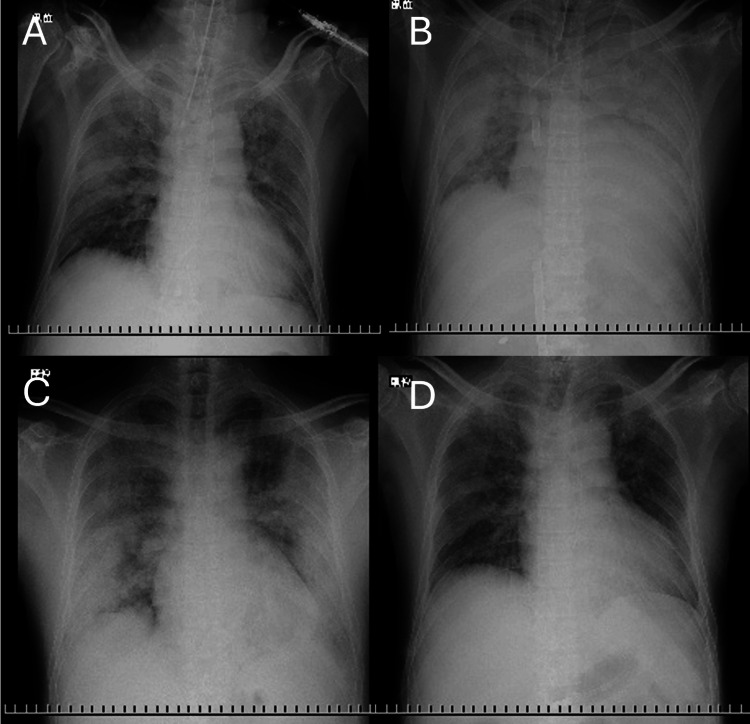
Serial chest X-rays from admission to transfer. (A) Immediately after admission during mechanical ventilation. (B) At the time of V-V ECMO initiation. (C) After weaning from V-V ECMO. (D) Upon transfer to our department, showing residual diffuse bilateral opacities.
